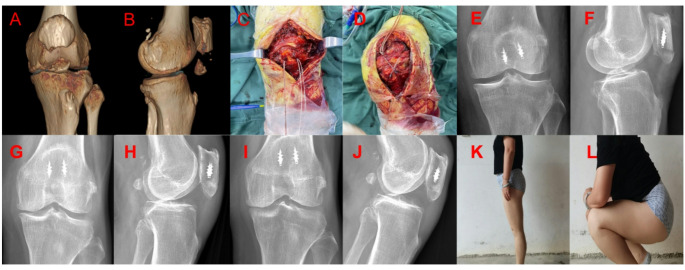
Clinical example. (A, B) Preoperative three-dimensional CT, (C, D) Intraoperative pictures, (E, F) Postoperative anteroposterior and lateral X-rays, (G, H) Postoperative anteroposterior and lateral X-rays 3 months, (I, J) Postoperative anteroposterior and lateral X-rays 6 months, (K, L) Postoperative functional photos
